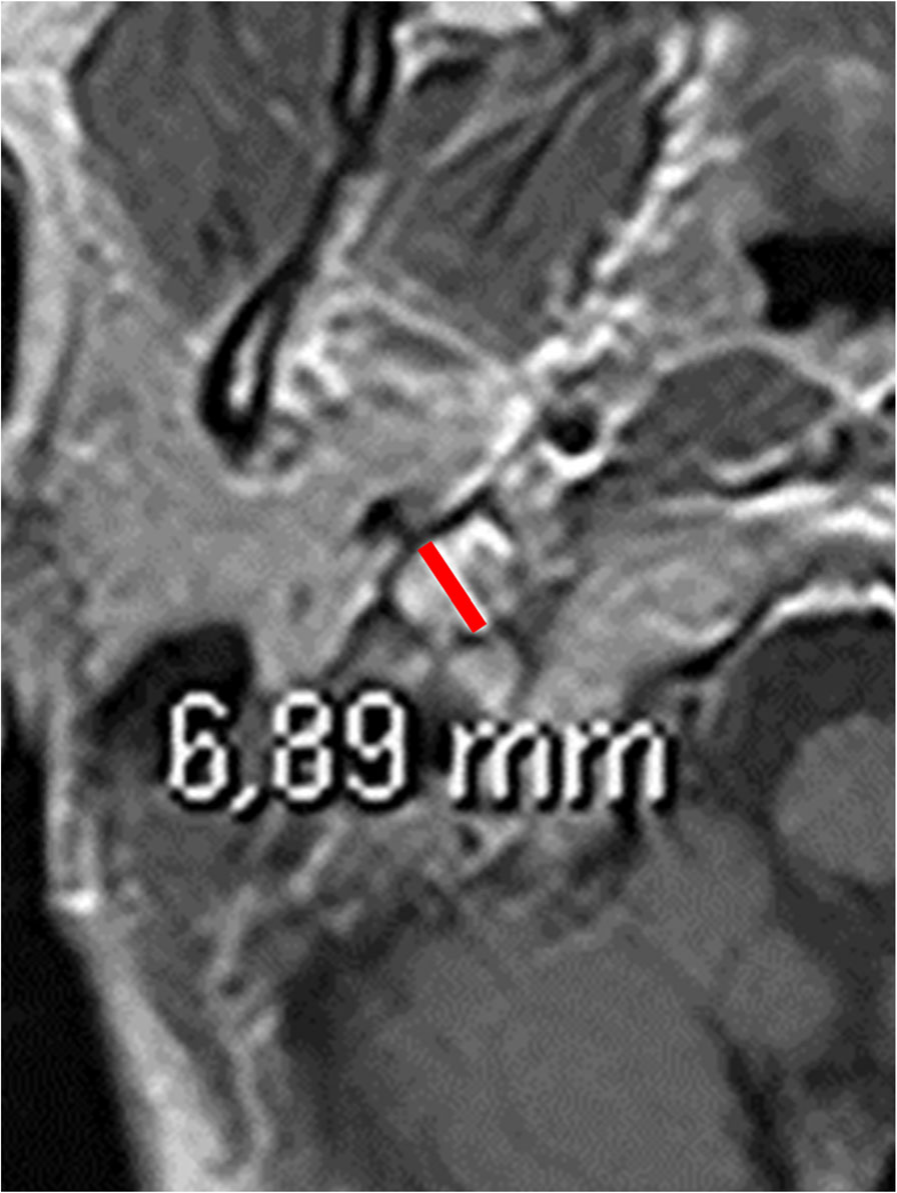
Magnification of a contrast-enhanced T1-weighted axial magnetic resonance image showing the method used to measure the jugular foramen diameter. Here the *red* caliper indicates the minimal diameter of the right jugular foramen
